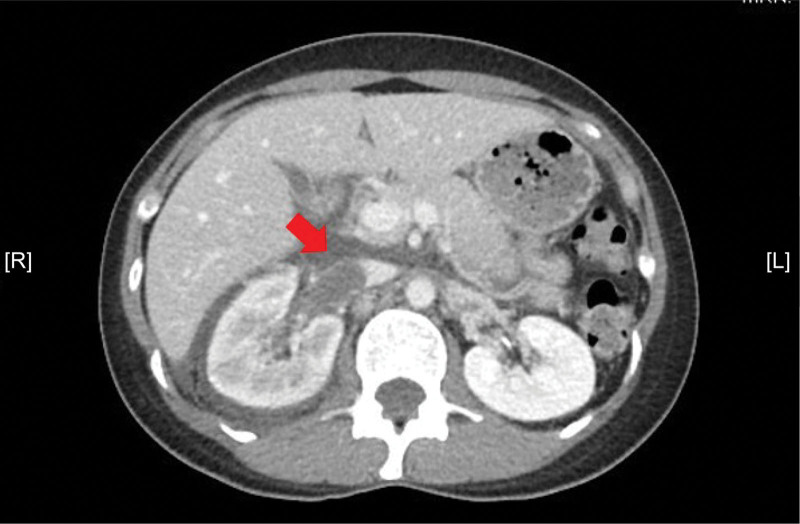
Contrasted abdominal CT images showing thrombi within the right renal veins and inferior vena cava. CT = computed tomography.
